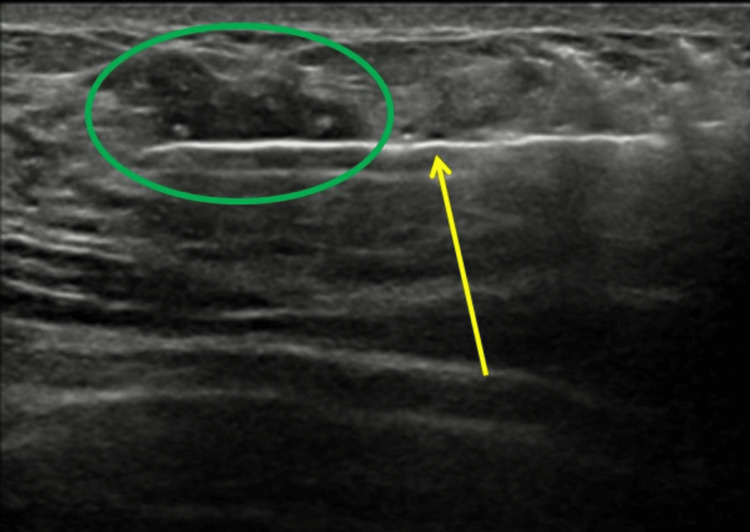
The suspicious irregular lesion within the left breast's lower inner quadrant (green circle) was targeted with an ultrasound-guided core biopsy needle (yellow arrow).
